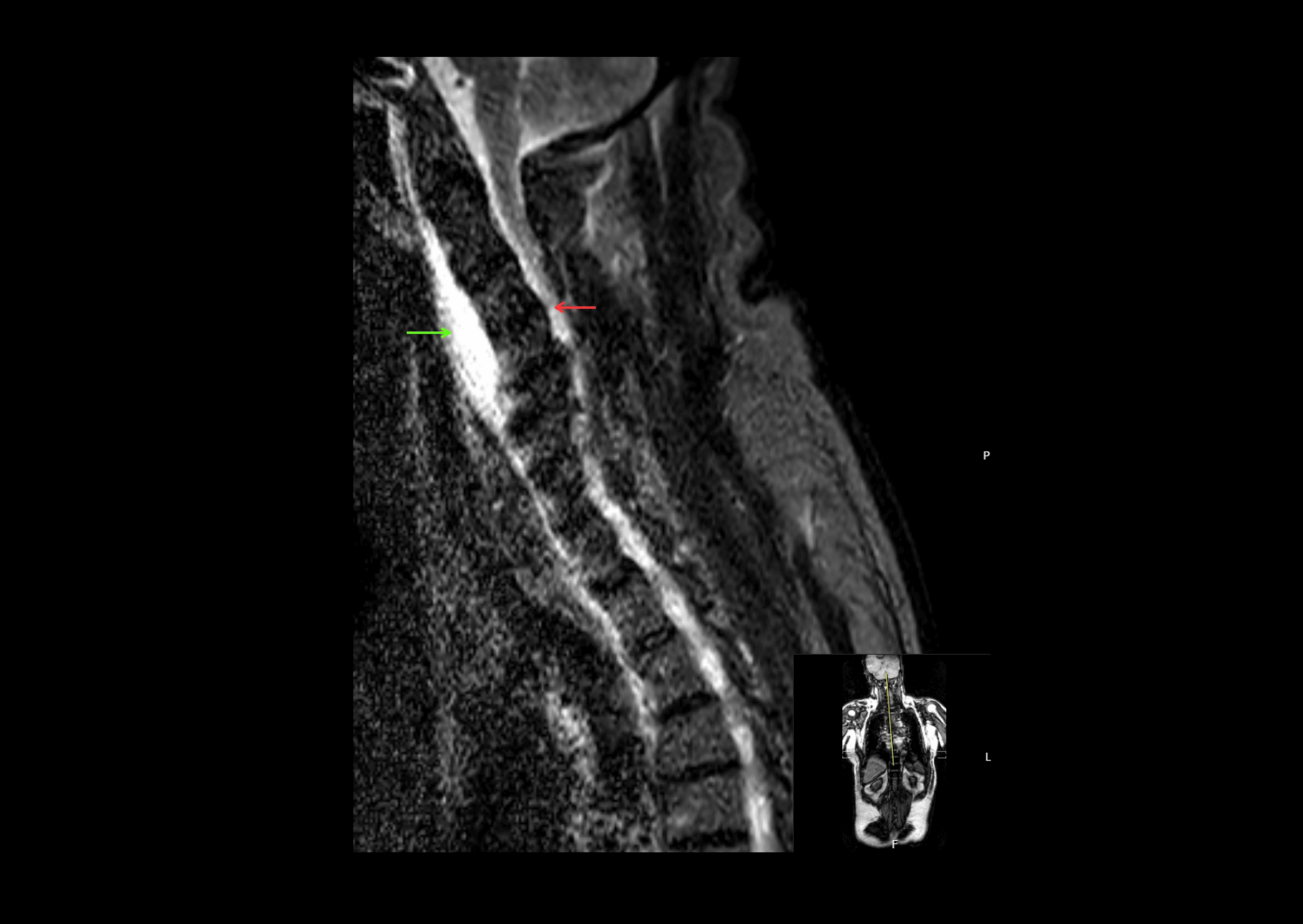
Sagittal T2-weighted MRI of the cervical spine The image demonstrates significant multilevel cervical spondylosis with loss of the normal cervical lordosis and associated spinal canal stenosis, characteristic of a central cord predominant injury pattern. The green arrow indicates a long-segment, poorly demarcated area of intramedullary T2-hyperintensity extending from the C3 to C6 vertebral levels, consistent with severe acute traumatic cord contusion and significant vasogenic edema. The red arrow points to a localized region of hyperintense signal within the posterior ligamentous complex and paraspinal soft tissues between the C3 to C6 vertebral levels; this finding is indicative of ligamentous strain or soft tissue signature typical of a high-energy hyperextension mechanism of injury MRI: magnetic resonance imaging
